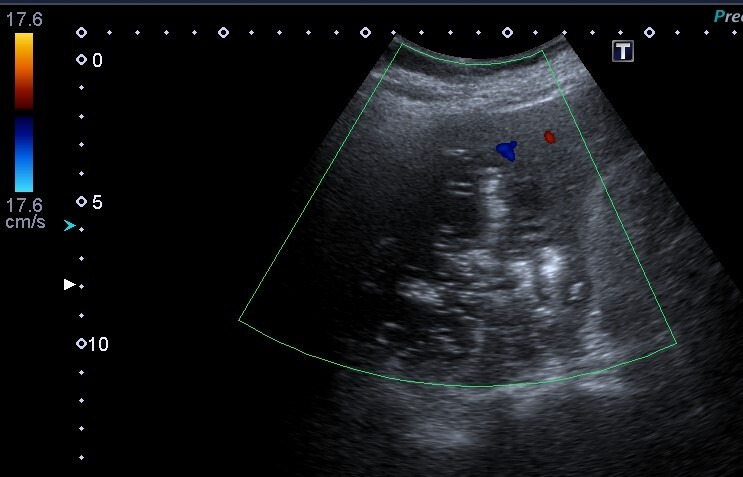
GFPLA with colour doppler, demonstrating no colour doppler signal within indicating the absence of centralperfusion
